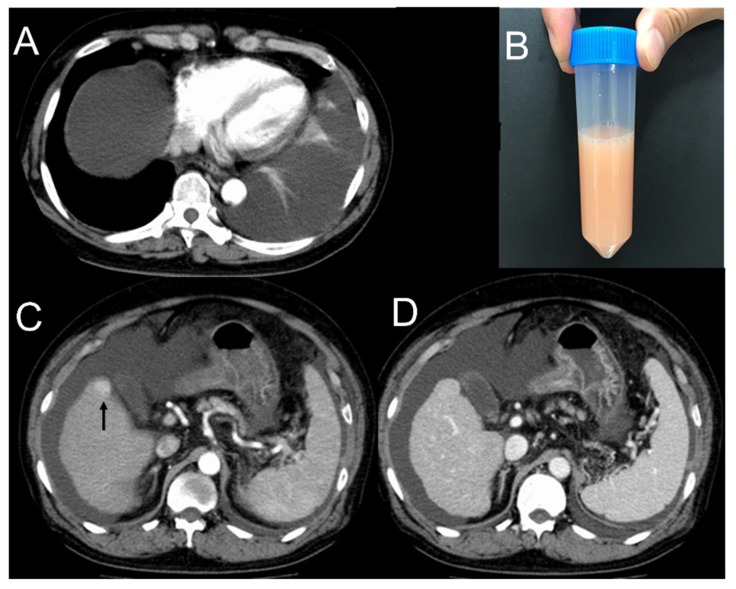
Imaging of chylothorax and computed tomography. (A) Computed tomography showed left pleural effusion and ascites. (B) The color of pleural effusion obtained by thoracentesis was milky white. (C,D) Contrast-enhanced computed tomography showed a 2-cm mass in the liver S6 that was enhanced in the arterial phase and washed out in the portal phase.
